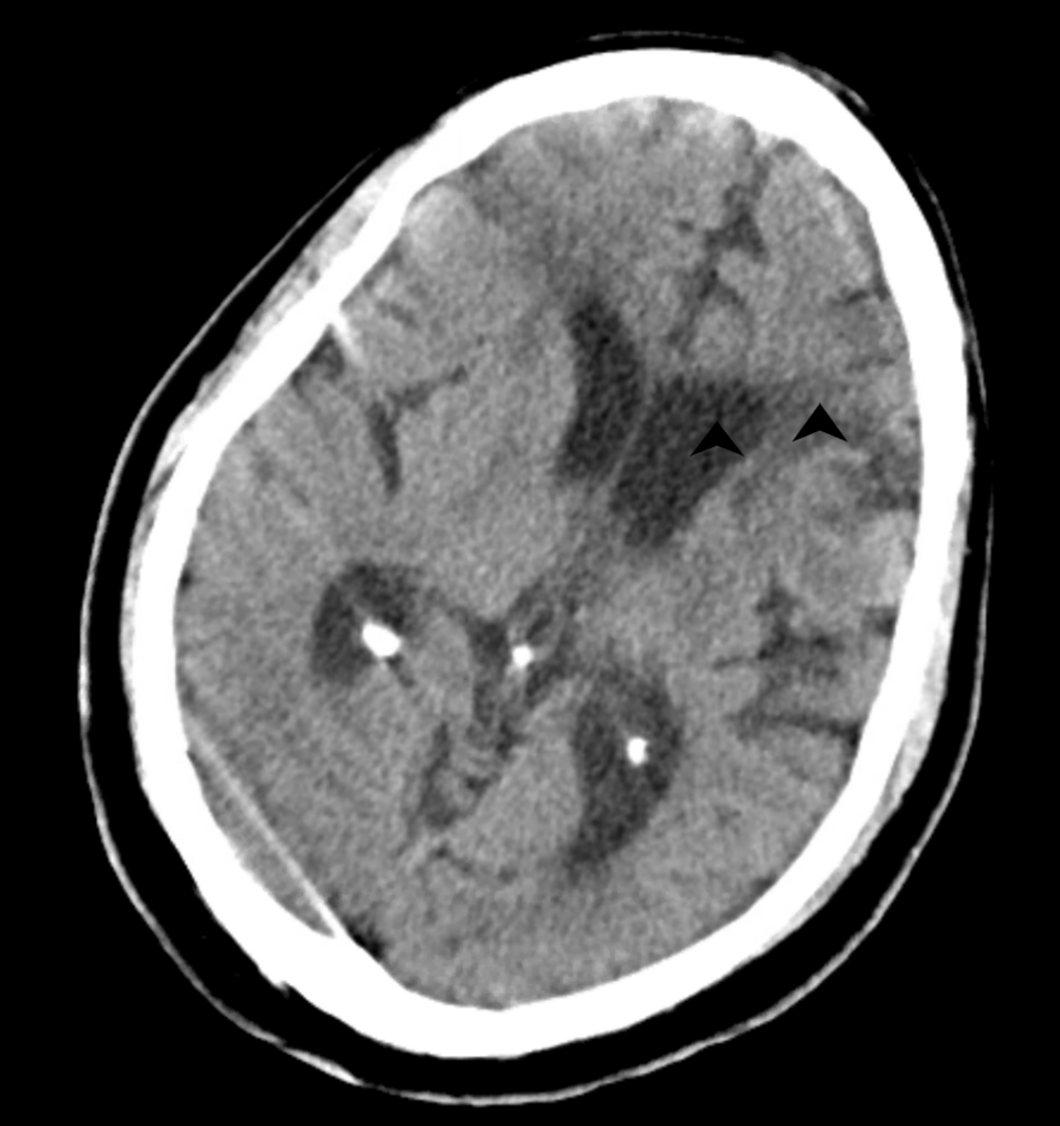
Non-contrast CT scan of the head showing enlarged left lateral ventricle and encephalomalacia in the left middle cerebral artery territory (black arrows).
